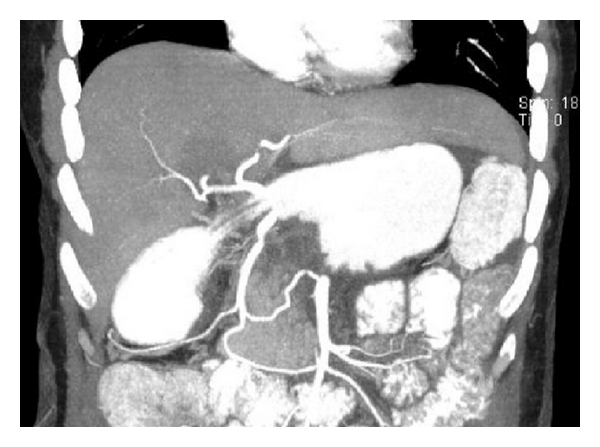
The postoperative arterial contrast-enhanced CT scan shows an excellent arterial flow to the hepatobiliary system via gastroduodenal artery and pancreatoduodenal arcades from the superior mesenteric artery.
